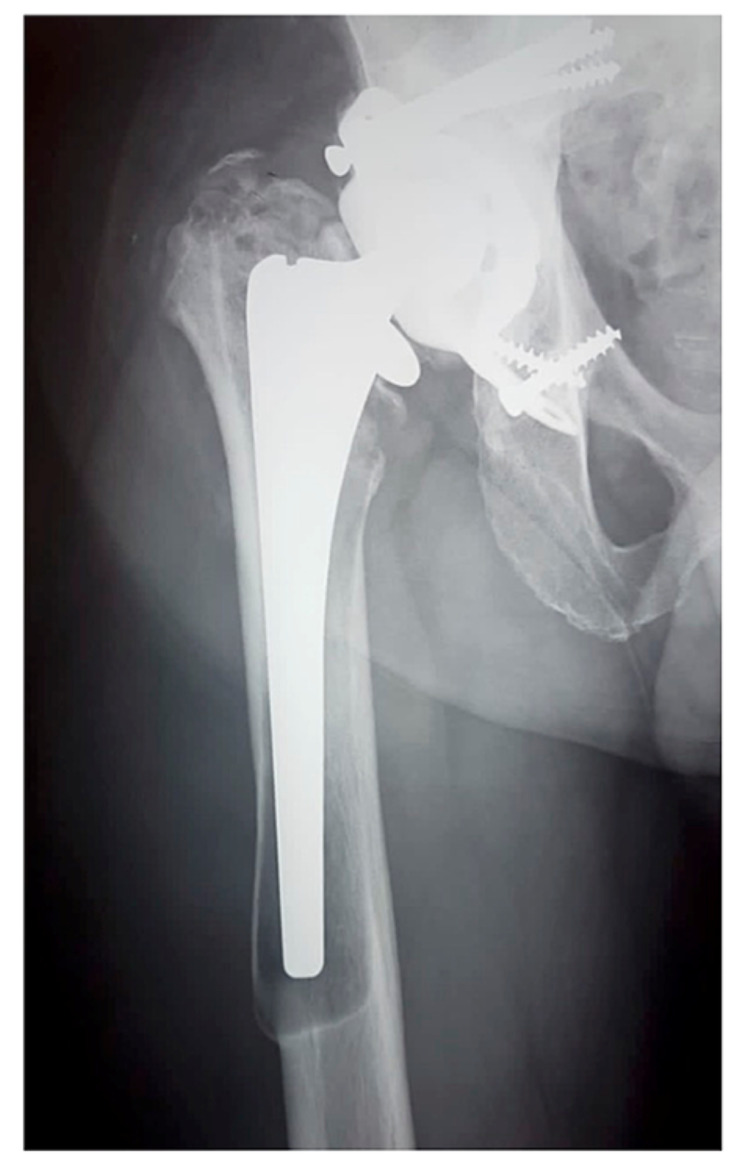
Radiograph showing progressive loosening of the femoral stem with distal migration and osteolysis at the tip of the stem with the risk of impending cortical perforation.
